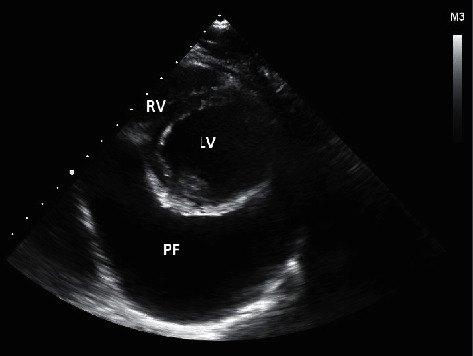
Echocardiography, short-axis view of the heart showing the LV and large pericardial effusion. LV: left ventricle; PF: pericardial fluid; RV: right ventricle.
